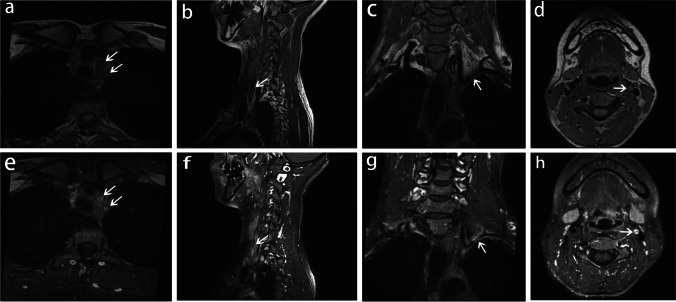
High-resolution MR images acquired from a clinical 3.0 Tesla system. T1-weighted spin-echo images (a-d) revealed vascular wall thickening, while gadolinium contrast-enhanced images (e–h) showed smooth concentric mural enhancement. (a) and (e), (b) and (f), (c) and (g), and (d) and (h) displayed the left CCA and the proximal left SCA in axial view, the left CCA in sagittal view, the left SCA in coronal view, and the left ICA in axial view, respectively
